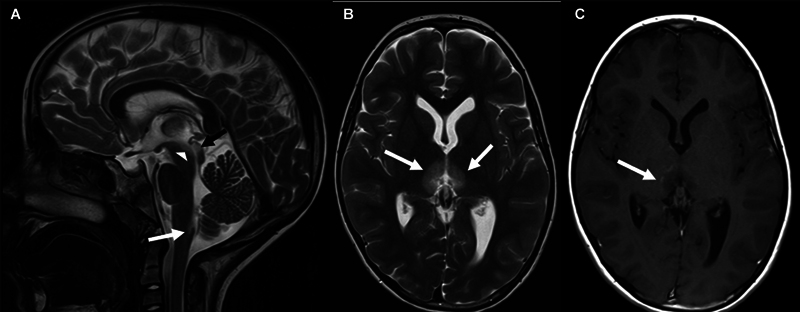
Initial brain magnetic resonance imaging (
